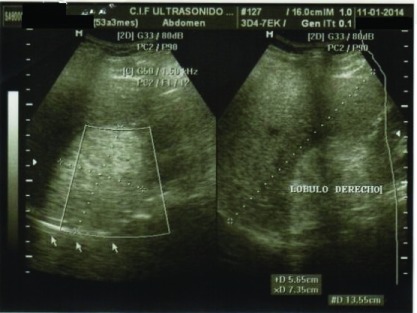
Abdominal ultrasound of the patient. A hyperechogenic 5.6 × 7.3 cm anchor is observed in segment V of the right hepatic lobe suggestive of an incidental hemangioma.
